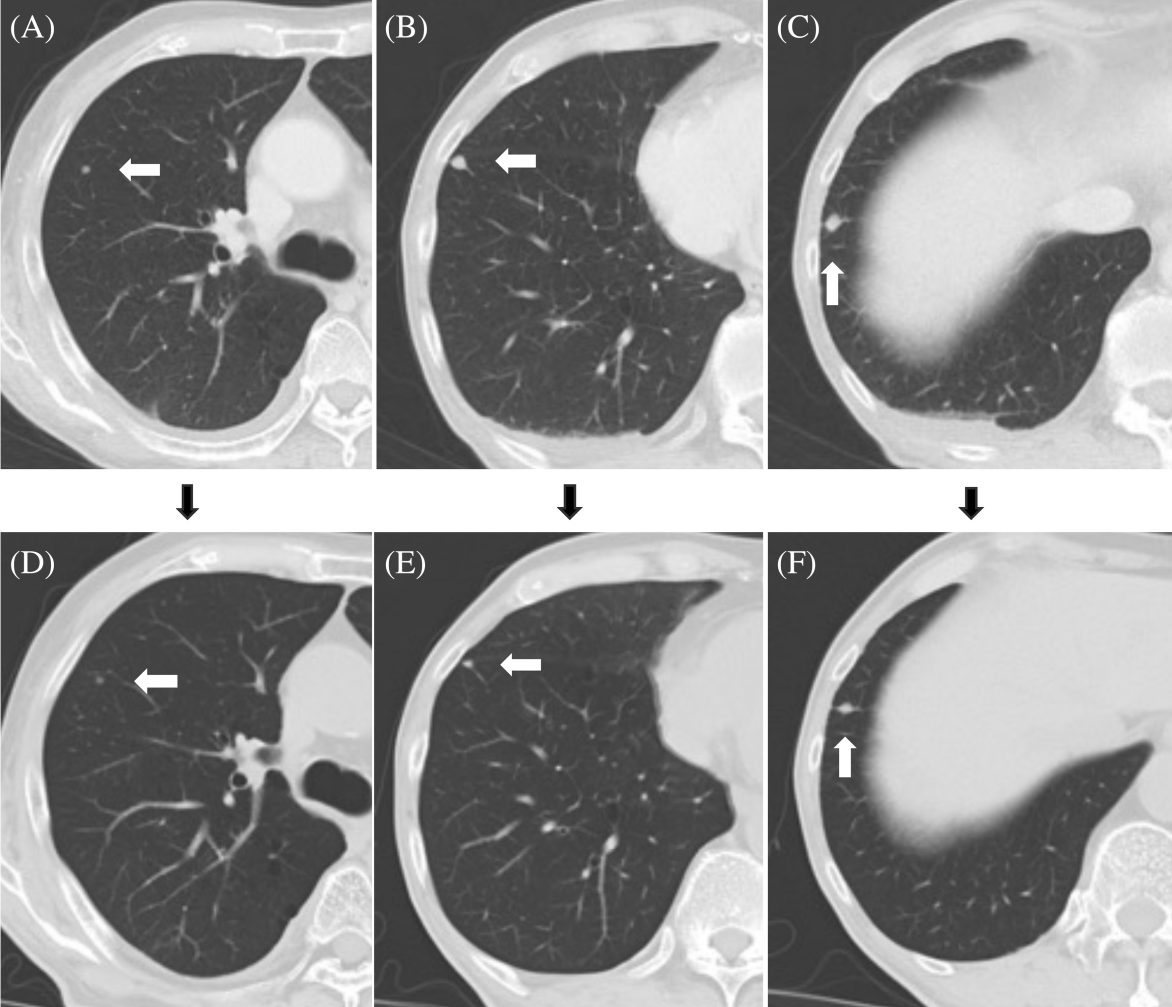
Computed tomography imaging (A–C) before and (D–F) after radiation. Lung metastases observed before radiotherapy are slightly reduced by radiotherapy of the primary tumor.
